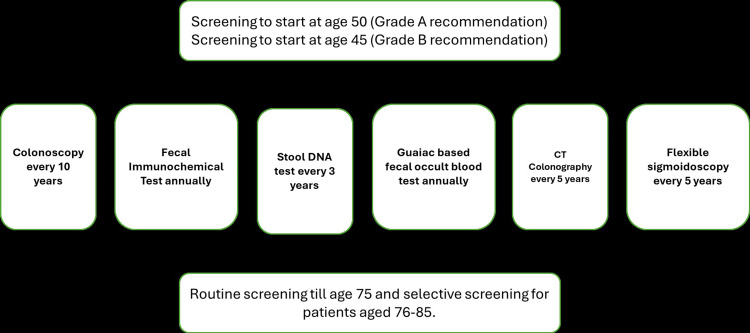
US Preventative Services Task Force (USPSTF) guidelines for colorectal cancer screening including direct and indirect modalities. Image credit - Hari Prasad Naidu Boyapati DNA - Deoxyribonucleic Acid; CT - Computed Tomography
